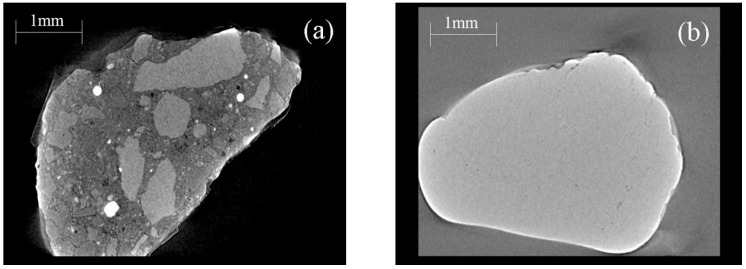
Computed tomography (CT) images of fine aggregate: (a) RFA and (b) NFA.
